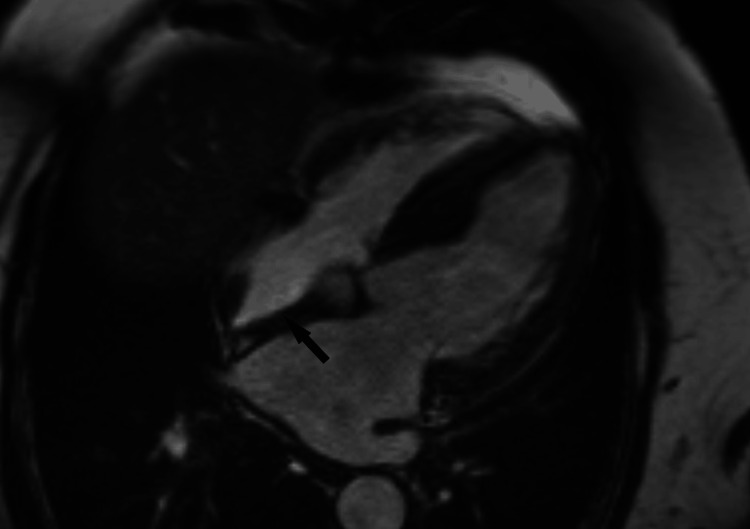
Cardiac magnetic resonance tomography T2-weighted images axial scan of the heart: Arrow shows hyperintense areas within the myocardium
